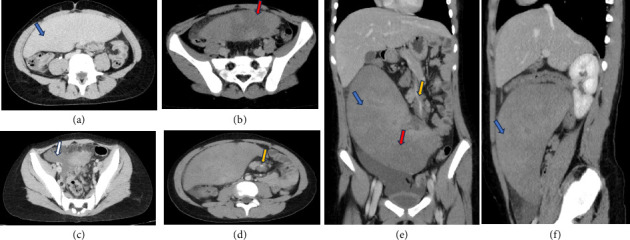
Abdomen CT with IV contrast in multiple planes, demonstrated: Absent spleen in the left upper quadrant; (a, e, f) massive enlarged spleen in the mid and lower abdomen (blue arrow); (b, e) hypodense heterogenous areas at the parenchyma of the spleen (red arrow) representing hypoperfusion, (d, e) whirled appearance of splenic vessels (yellow arrow); (c) pelvic free fluid (white arrow).
